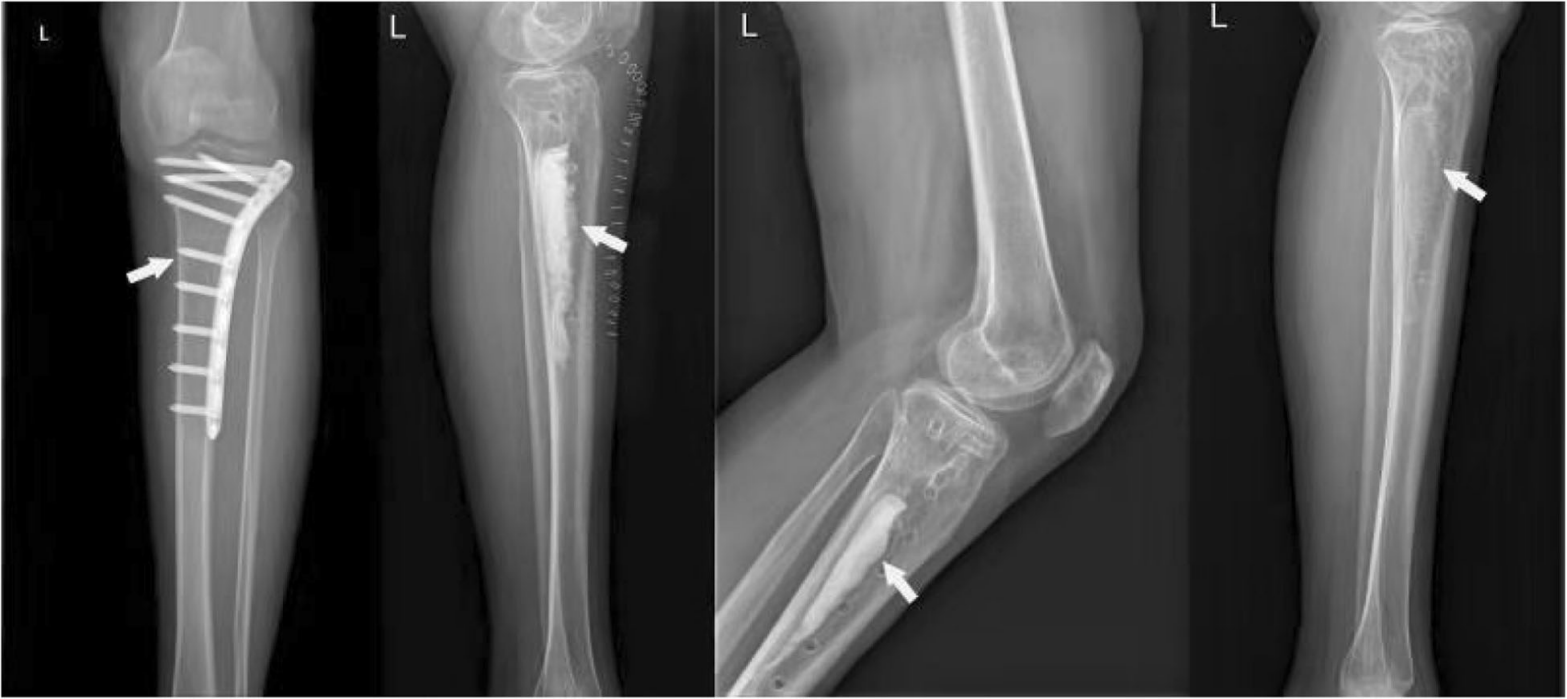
A patient with C-M type III tibial osteomyelitis received internal fixation removal and infected bone debridement, followed by implantation of antibiotic-loaded calcium sulfate. X-ray examination during the follow up showed dead space was generally substituted by new bone tissues
